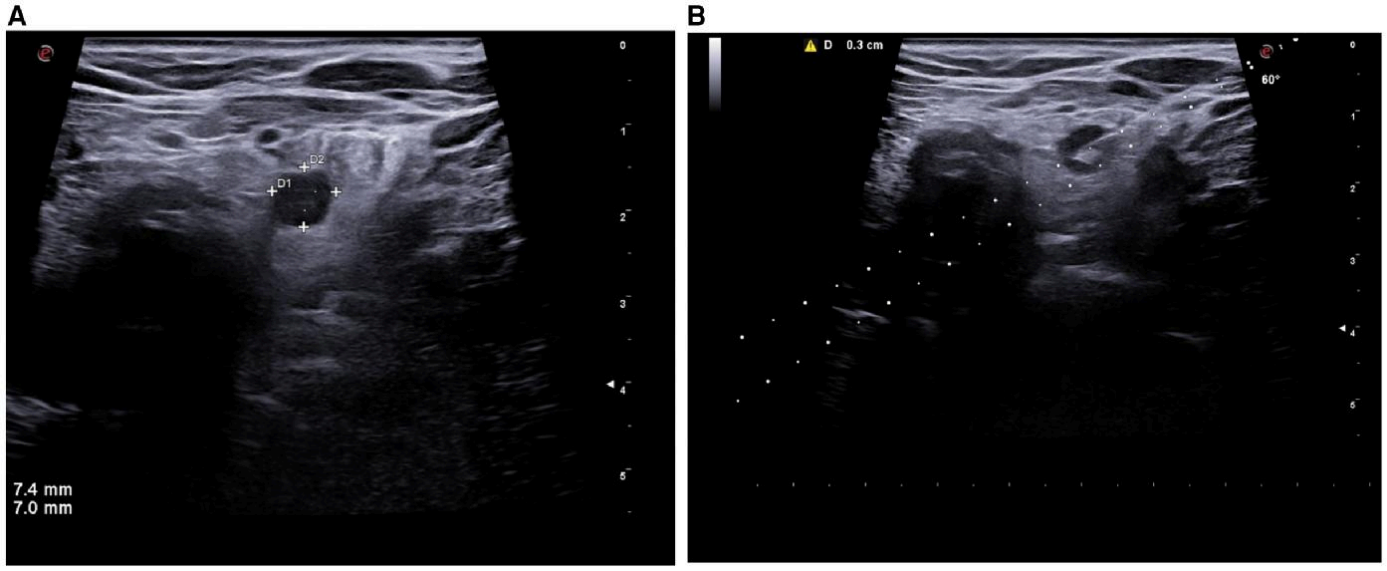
(A-B) Ultrasound image of voluminous nodule of the left thyroid lobe with regular margins and mixed solid and predominantly cystic component with colloid-hematic content.
